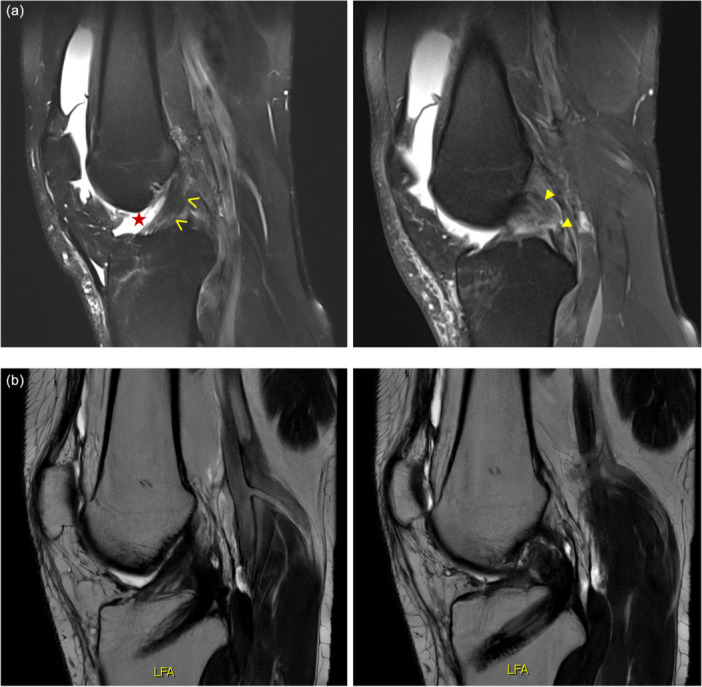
Sagittal MR images of a patient with a PCL injury. (a) Fat‐suppressed T2‐weighted MR images obtained prior to surgery: depiction of a positive ‘empty roof sign’ (★) with a sagging and undulating configuration of the ACL (<); the PCL is fully ruptured (►). (b) T2‐weighted MR images obtained after PCL reconstruction surgery: the ‘empty roof sign’ is negative and the PCL is reconstructed. ACL, anterior cruciate ligament; MR, magnetic resonance; PCL, posterior cruciate ligament.
